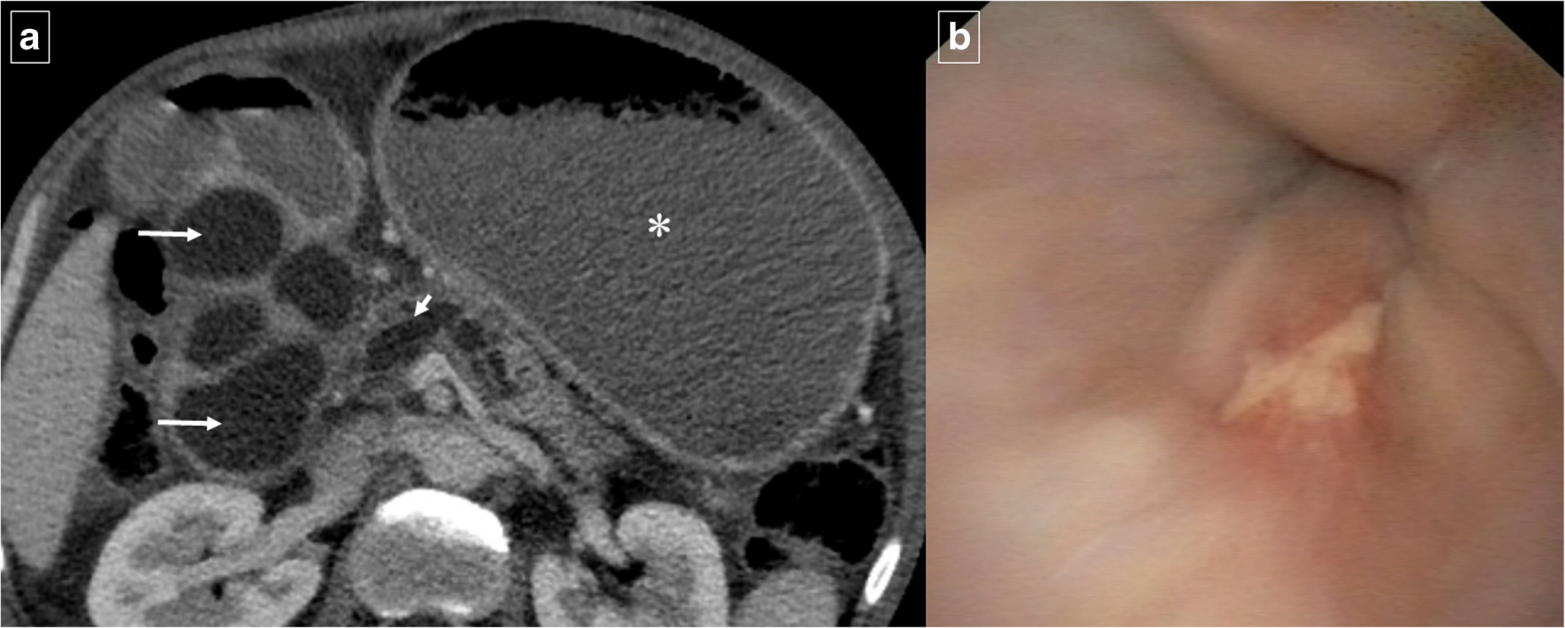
A 56-year-old man with history of alcohol abuse and smoking presented with weight loss. a Axial contrast-enhanced CT reveals cystic dystrophy with multiple cystic parietal areas (arrows) in the thickened second duodenal portion causing severe duodenal obstruction with gastric dilation (*). Atrophy of the pancreatic parenchyma and strictures of the pancreatic duct (short arrow) due to chronic pancreatitis. b Detail from the upper gastrointestinal endoscopy study showing extrinsic compression of the duodenal lumen
